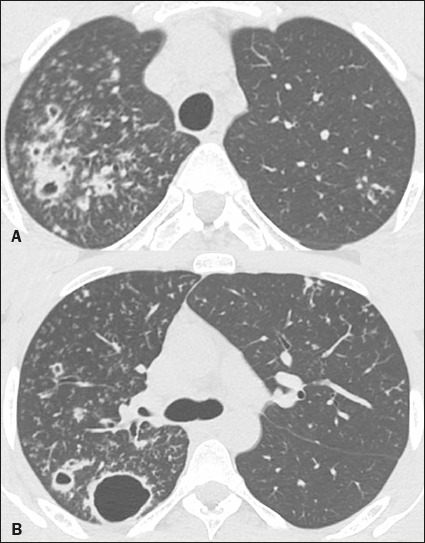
A 22-year-old man with COVID-19 and tuberculosis. Chest CT showing
